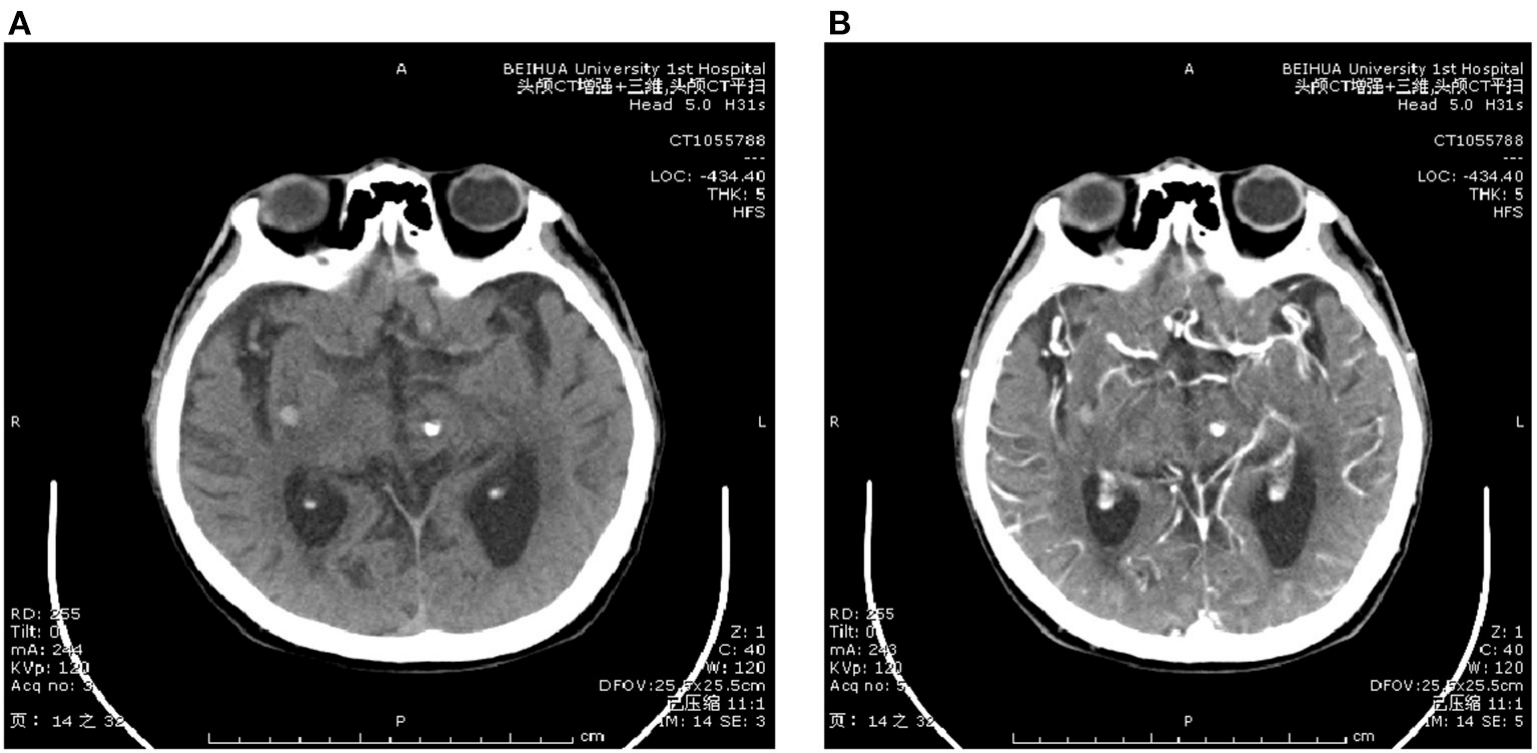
CT scanning image. (A) Shows CT plain scan image and (B) shows CT enhanced image.
